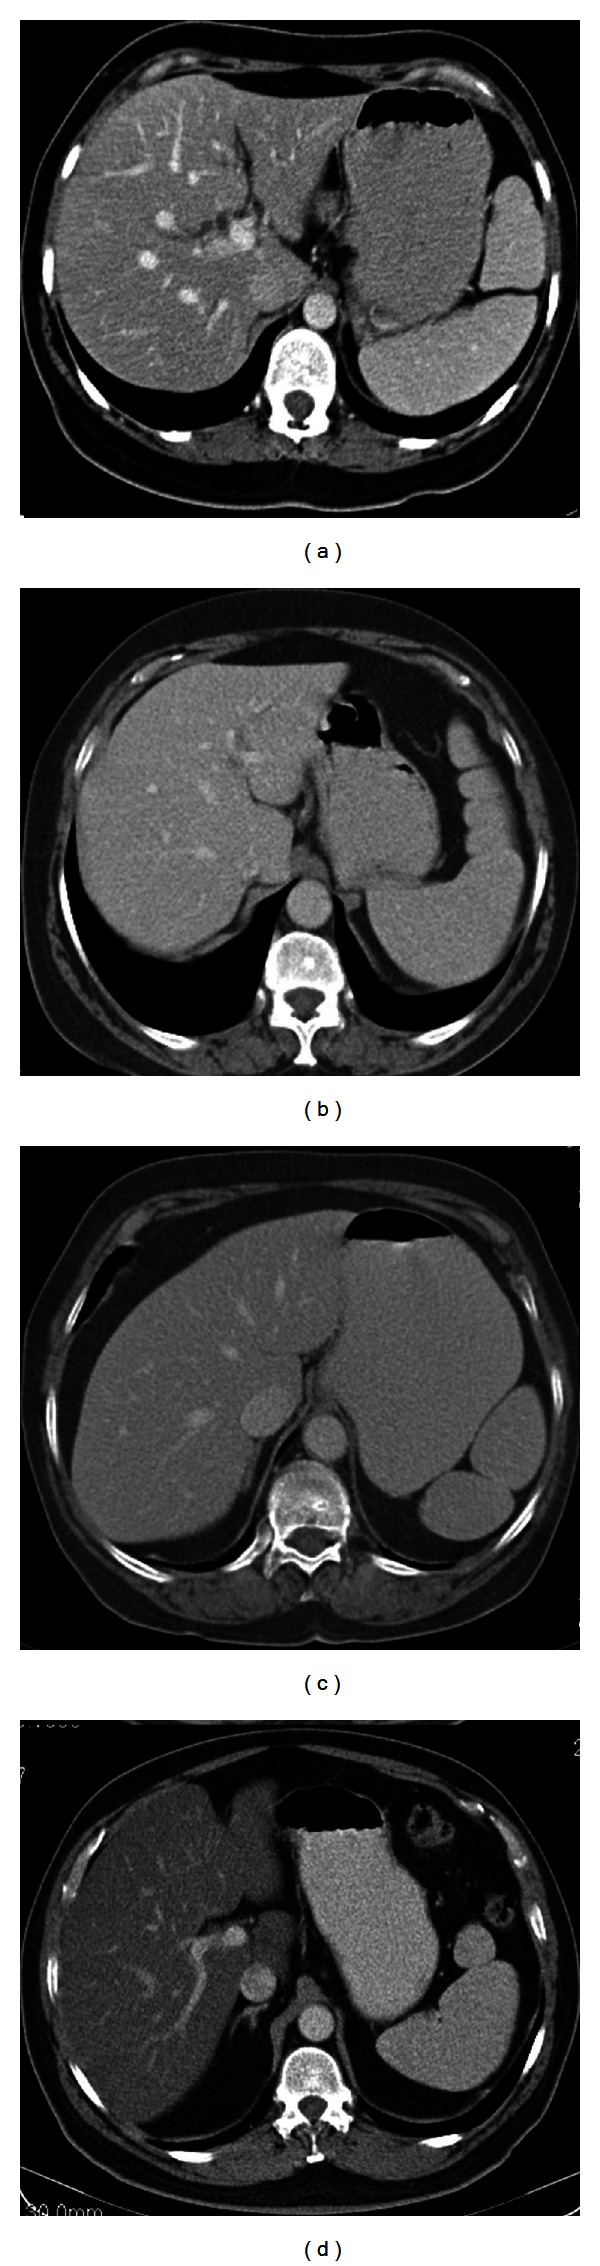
Axial contrast enhanced CT images show examples of splenic clefts in four different patients which were incidentally detected. In (b) there are also coexisting fetal lobules at the medial aspect of the spleen.
